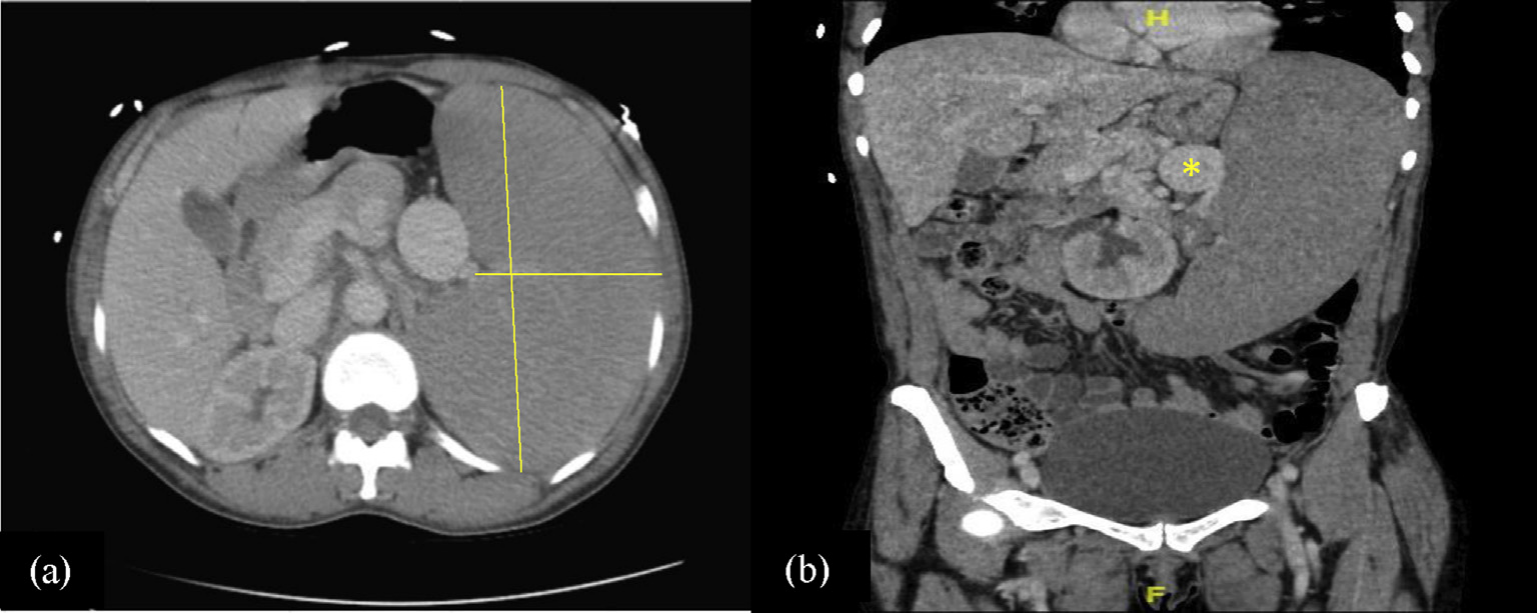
(a) CT abdomen and pelvis with contrast demonstrated marked splenomegaly, spleen measuring 25 cm craniocaudad, 8 cm transverse, 18.5 cm anterior-posterior. (b) Coronal view showing marked enlargement of the splenic veins, multiple splenic artery aneurysms and massive splenomegaly. Largest aneurysm was 6 cm.
